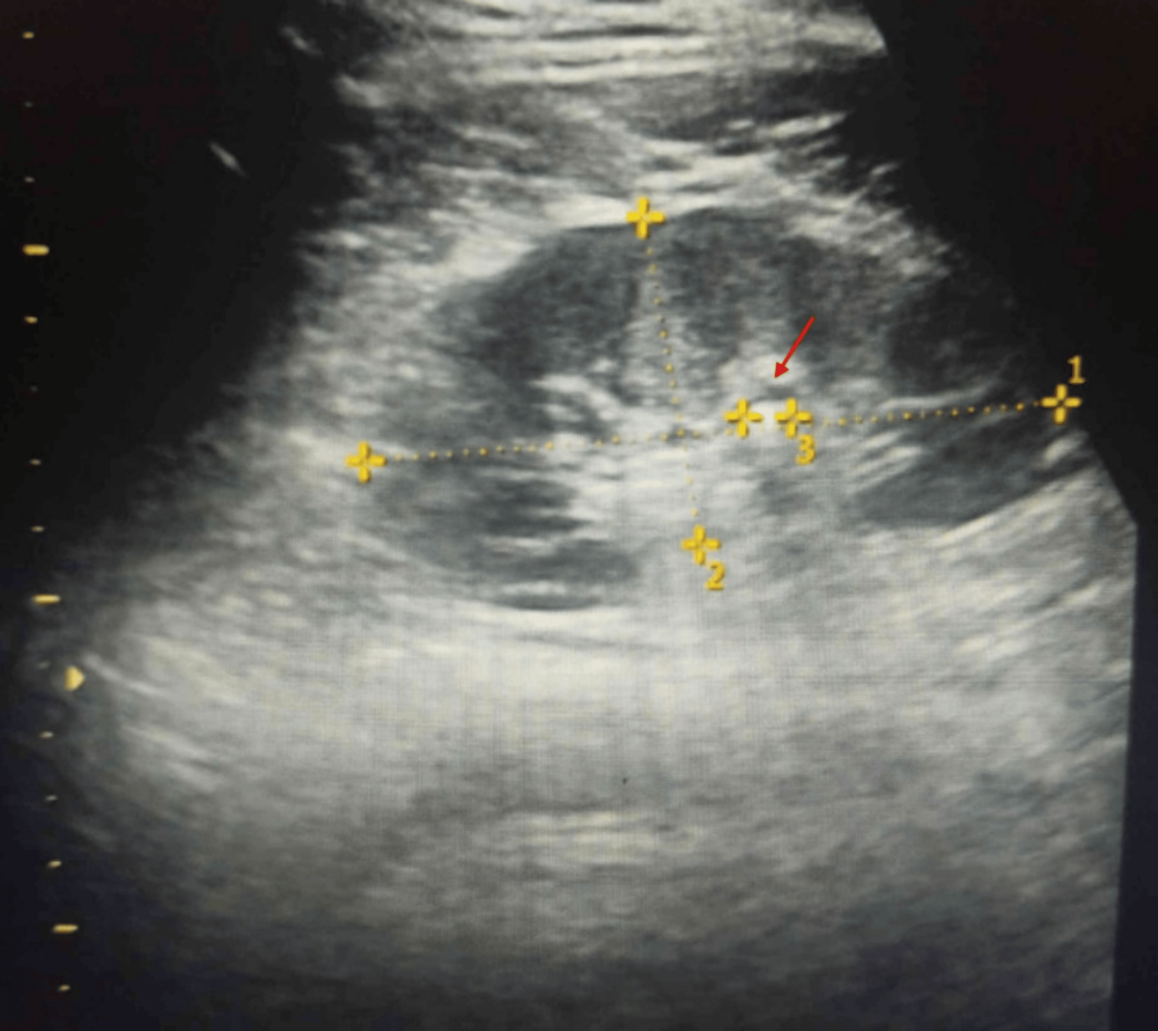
Ultrasound showing a 5 mm kidney stone. Red arrow showing a 5 mm stone.
